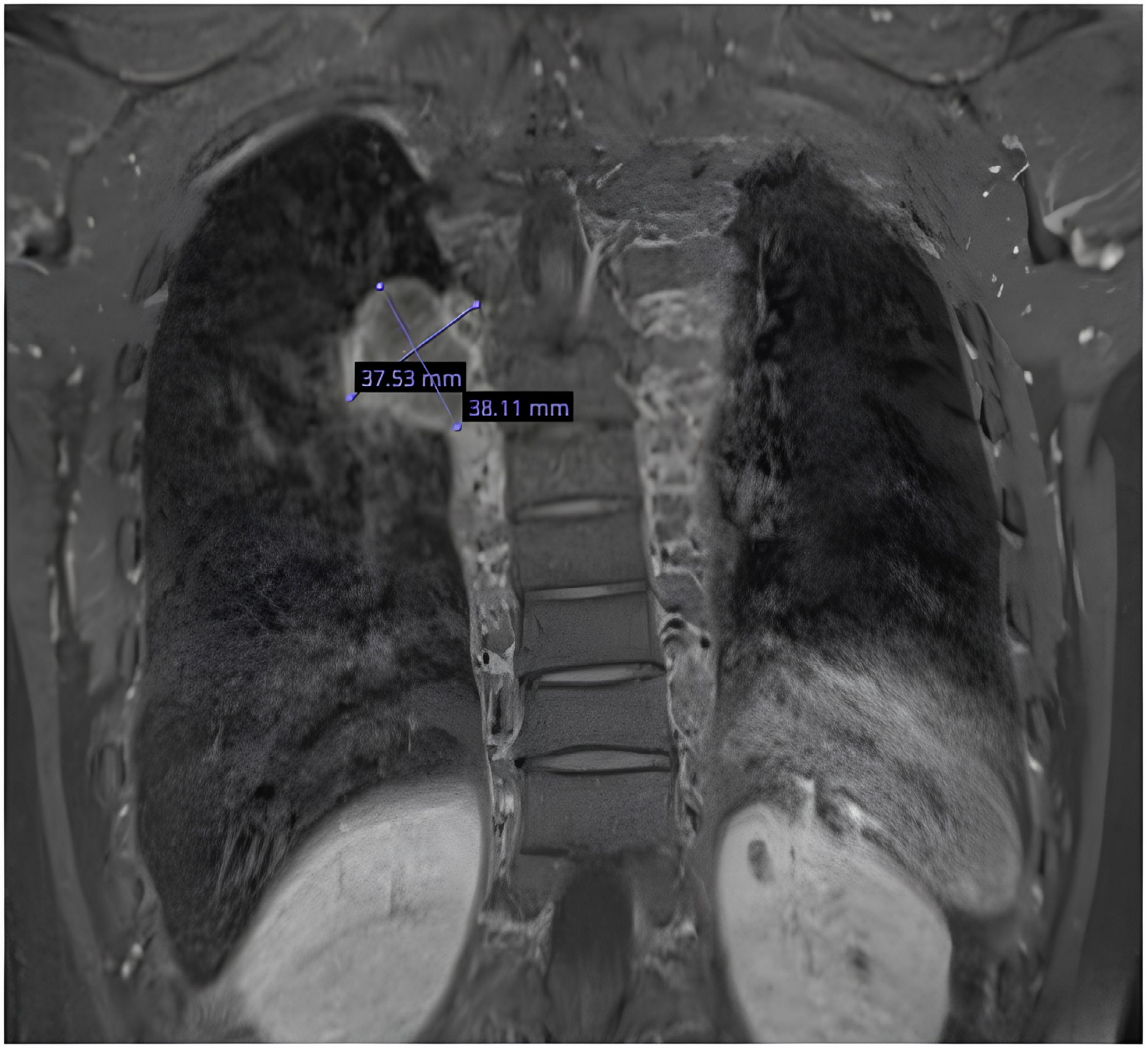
MRI thoracic spine dated April\2021.
